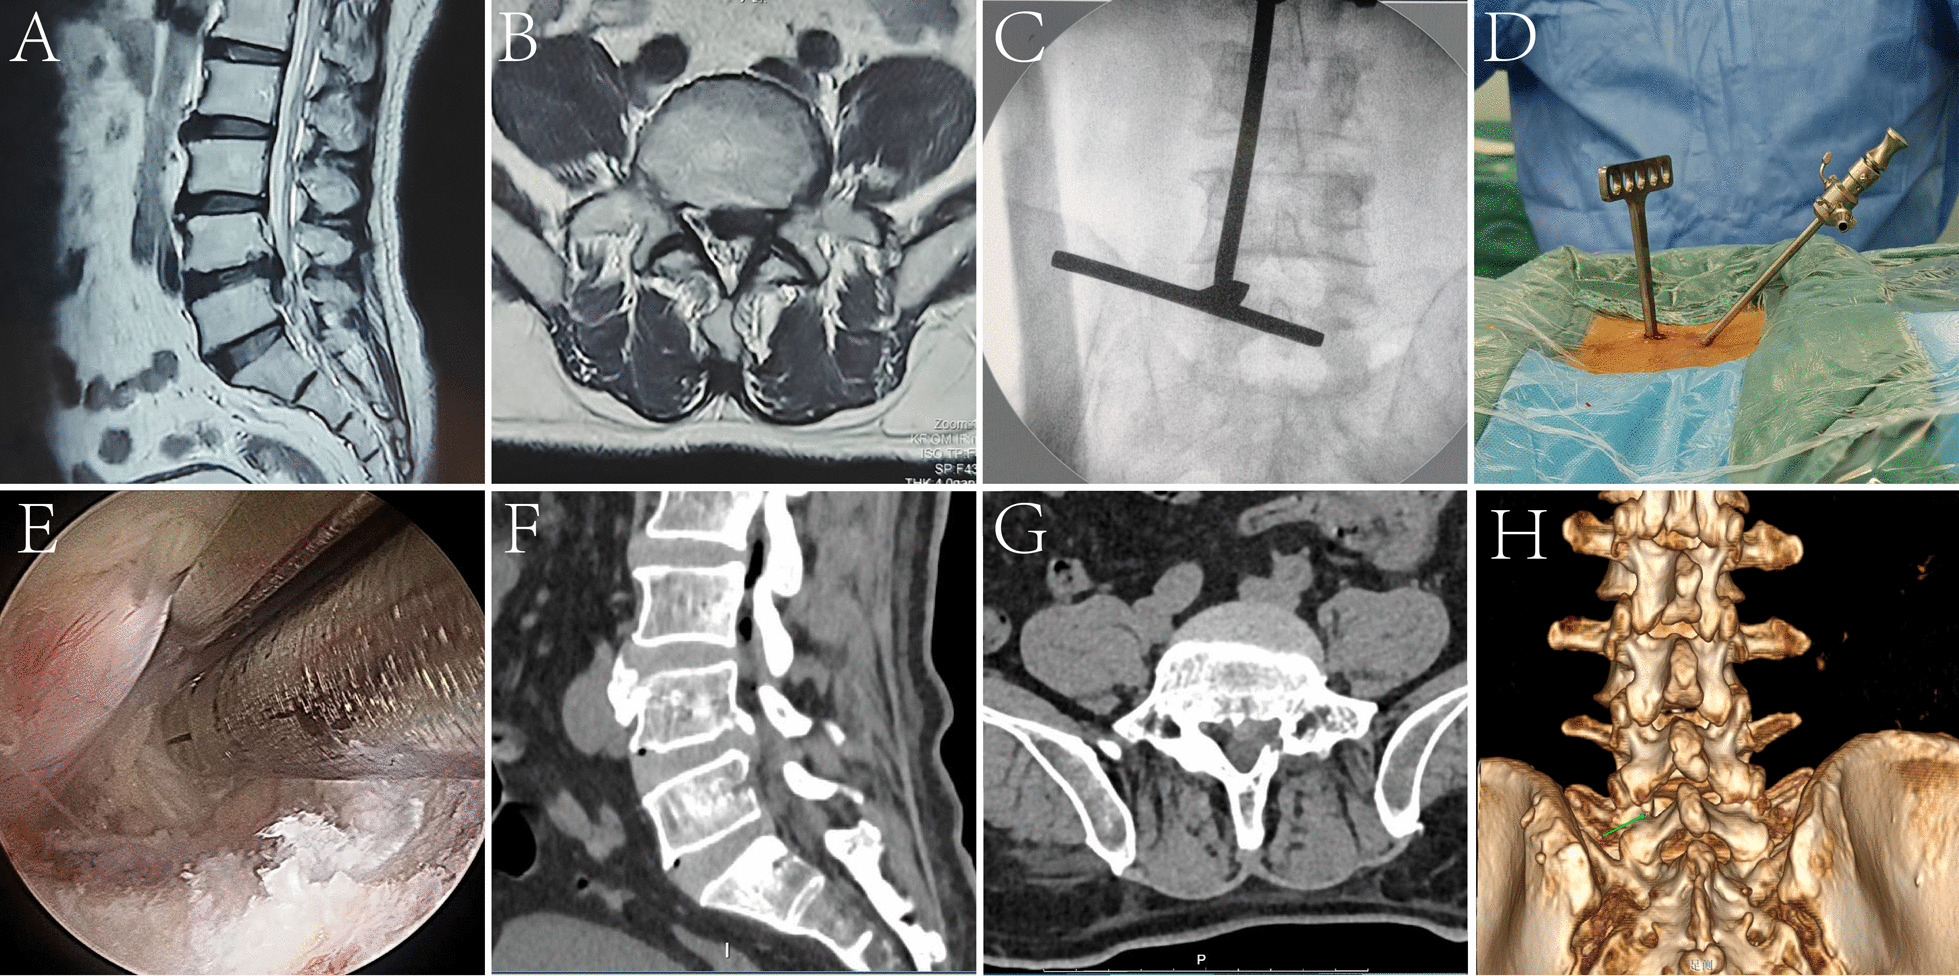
Surgical procedure of UBED and pre/postoperative imaging of patients with high-grade downward migrated LDH. A, B Preoperative sagittal and transverse MRI showed high-grade downward migration of the L4/5 disc. C–E Image C depicts the anteroposterior fluoroscopy utilized to guide the UBED procedure. Image D highlights the UBED working channel. Image E displays the decompressed nerve root following successful UBED. F–H Postoperative sagittal and coronal CT imaging of the operated area reveals the absence of any remaining migrated nucleus pulposus. Additionally, 3D reconstruction enables clear visualization of the defective bone
